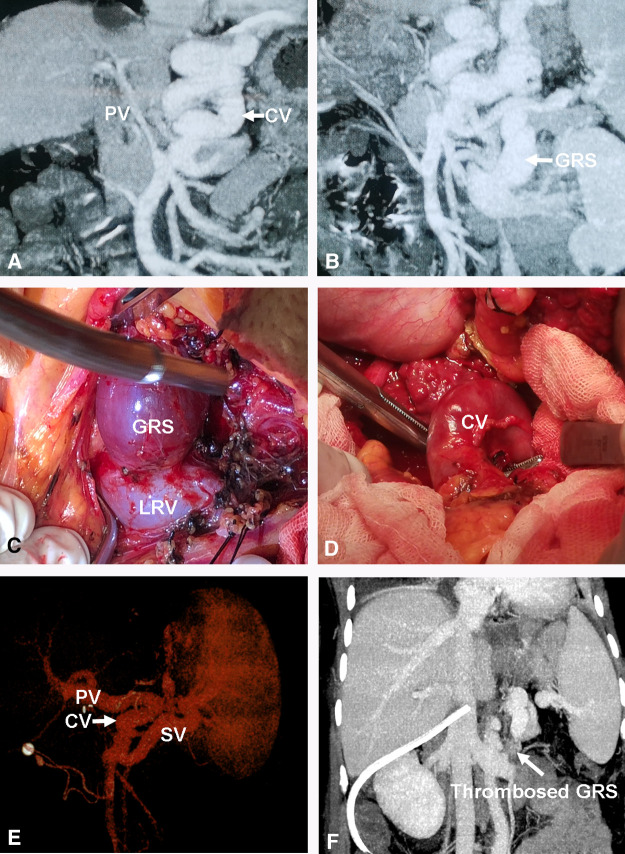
Direct ligation of a large GRS and reconstruction of gastric CV with PV. (A) Small diameter PV or cavernous transformation of PV. (B) Large GRS before transplantation. (C) Isolation of large GRS. (D) Isolation of gastric CV. (E) The images of the PV reconstruction with recipient gastric CV and graft PV on 3D CT. (F) Thrombosed GRS on day 7 post transplantation. CV, coronary vein; GRS, gastrorenal shunt.
